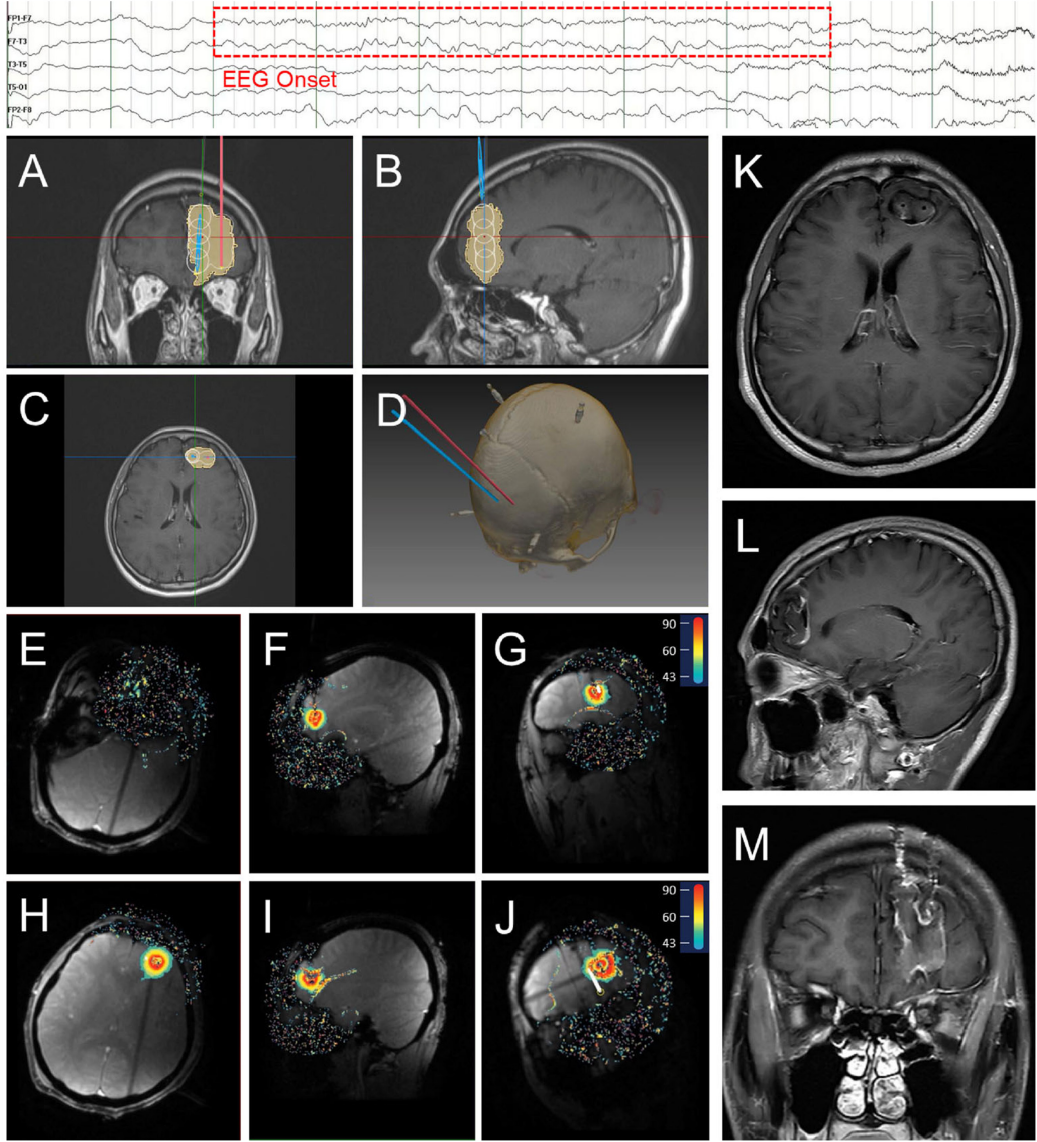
Representative FCD patient's preoperative ablation trajectory planning, intraoperative ablation real‐time thermal monitoring, and postoperative MRI. The top of the figure indicates EEG onset. (A–C) Ablation trajectory planning in coronal, sagittal, and axial plane. (White indicates the planned ablation and yellow indicates the actual ablation); (D) thin‐slice head CT with reconstruction; (E–G) ablation along the first trajectory in axial, sagittal, and coronal plane; (H–J) ablation along the second trajectory in axial, sagittal, and coronal plane; (K–M) postoperative 3‐month MRI in axial, sagittal, and coronal plane.
